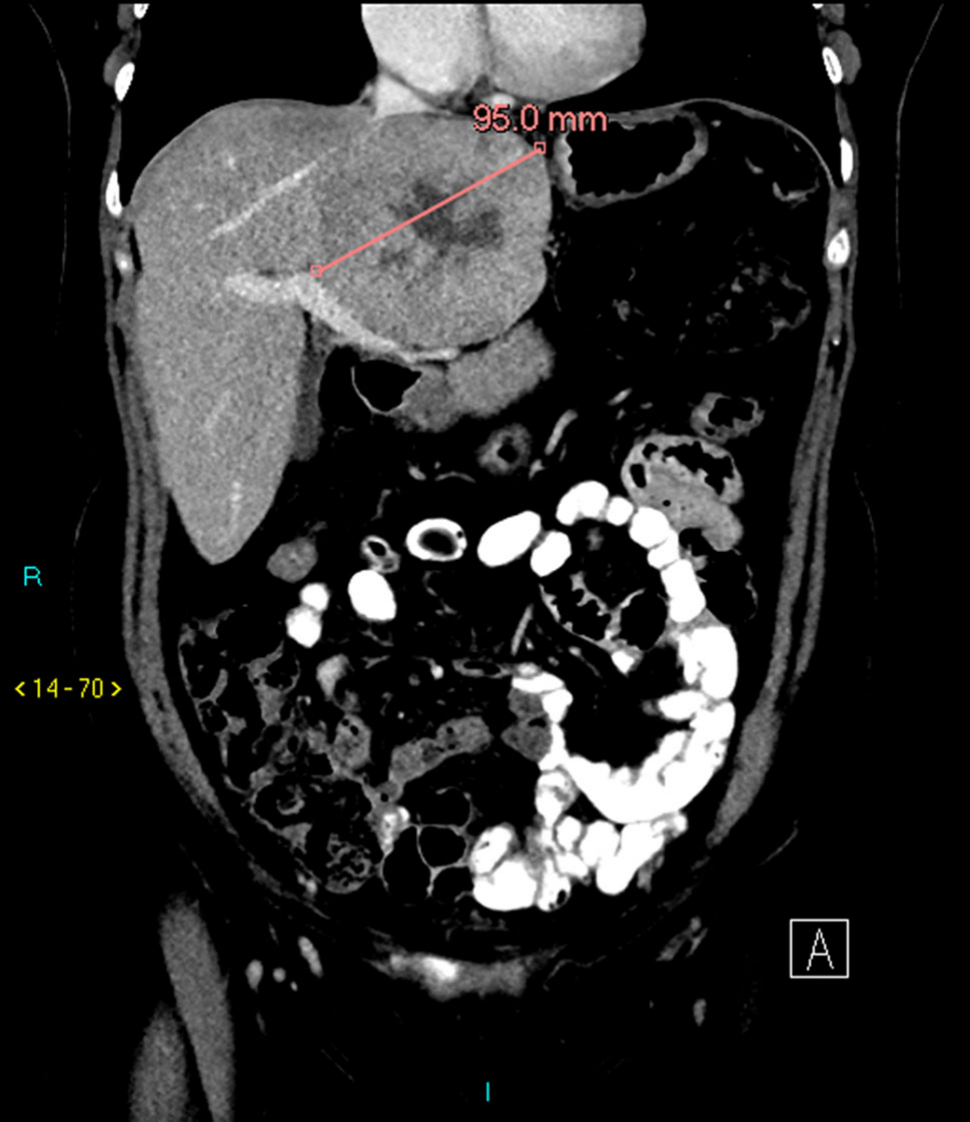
Coronal contrast-enhanced CT demonstrating a 9.5-cm (350 cm^3^) mass lesion centred in the caudate lobe but extending into segments 4a, 7 and 8
